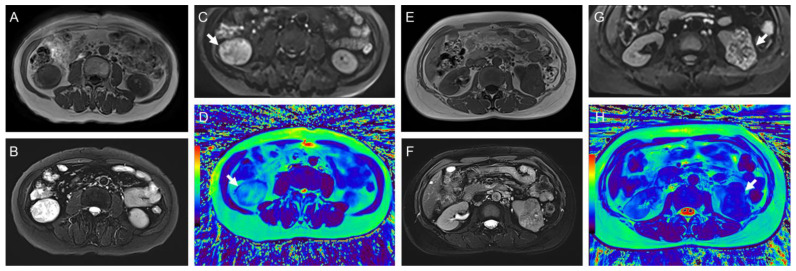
(A–D) Representative images of a 65-year-old woman with WHO/ISUP II ccRCC in the right kidney (arrow): (A) axial T1-weighted image; (B) T2-weighted image; (C) diffusion-weighted images (b = 800 s/mm^2^); (D) radial TSE T2 map. The mean ADC and T2 values of the tumor were 1.51 mm^2^/s and 141.4 ms, respectively. (E–H) Representative images of a 51-year-old man with WHO/ISUP III ccRCC with tumor necrosis in the left kidney (arrow): (E) axial T1-weighted image; (F) T2-weighted image; (G) diffusion-weighted images (b = 800 s/mm^2^); (H) radial TSE T2 map. The mean ADC and T2 values of the tumor were 1.11 mm^2^/s and 85.9 ms, respectively. The color bar in the pseudocolor images (D and H) represents T2 values, which gradually increase from black to red.
